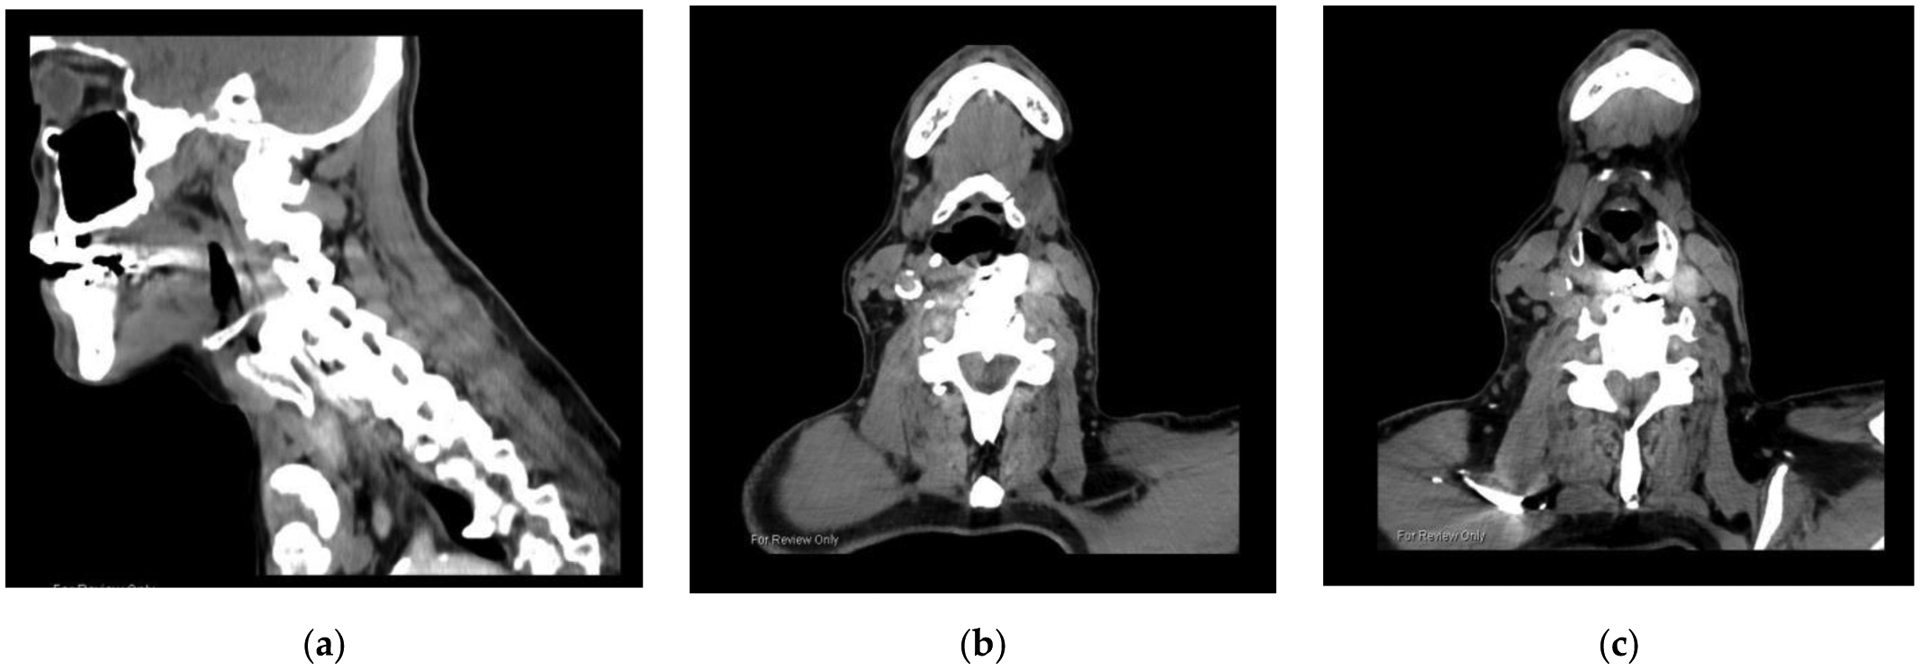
CT images show a large bony overgrowth fusing the larynx to anterior cervical spine: (a) sagittal view; (b) axial view of upper border at C3 level; (c) lower border of bony overgrowth at C6 level. Figure created by author.
